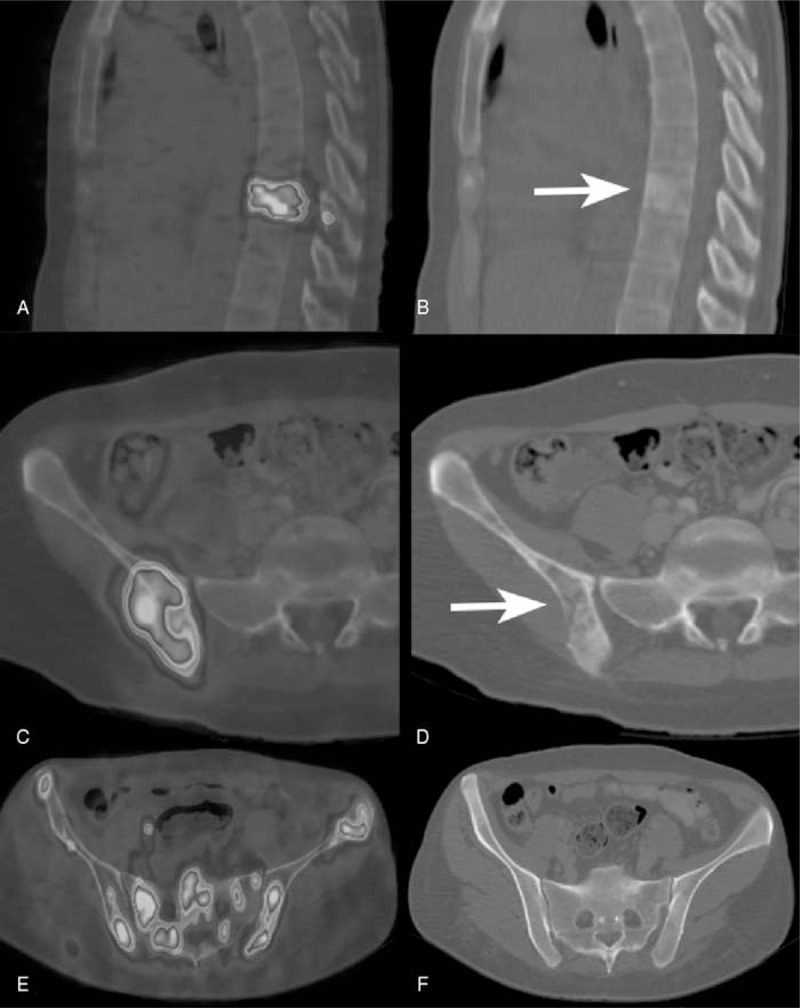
(A) Focal ^18^F FDG uptake in a thoracic vertebrae corresponding to a sclerotic lesion that appears hyperdense and irregular in ceCT (B). Increased ^18^F FDG uptake in the right ilium (C) corresponding to a lytic area (soft-tissue attenuation with irregular margins) in ceCT (D). Diffuse ^18^F FDG uptake in the pelvis (E) in the absence of morphological abnormalities in ceCT (F).
